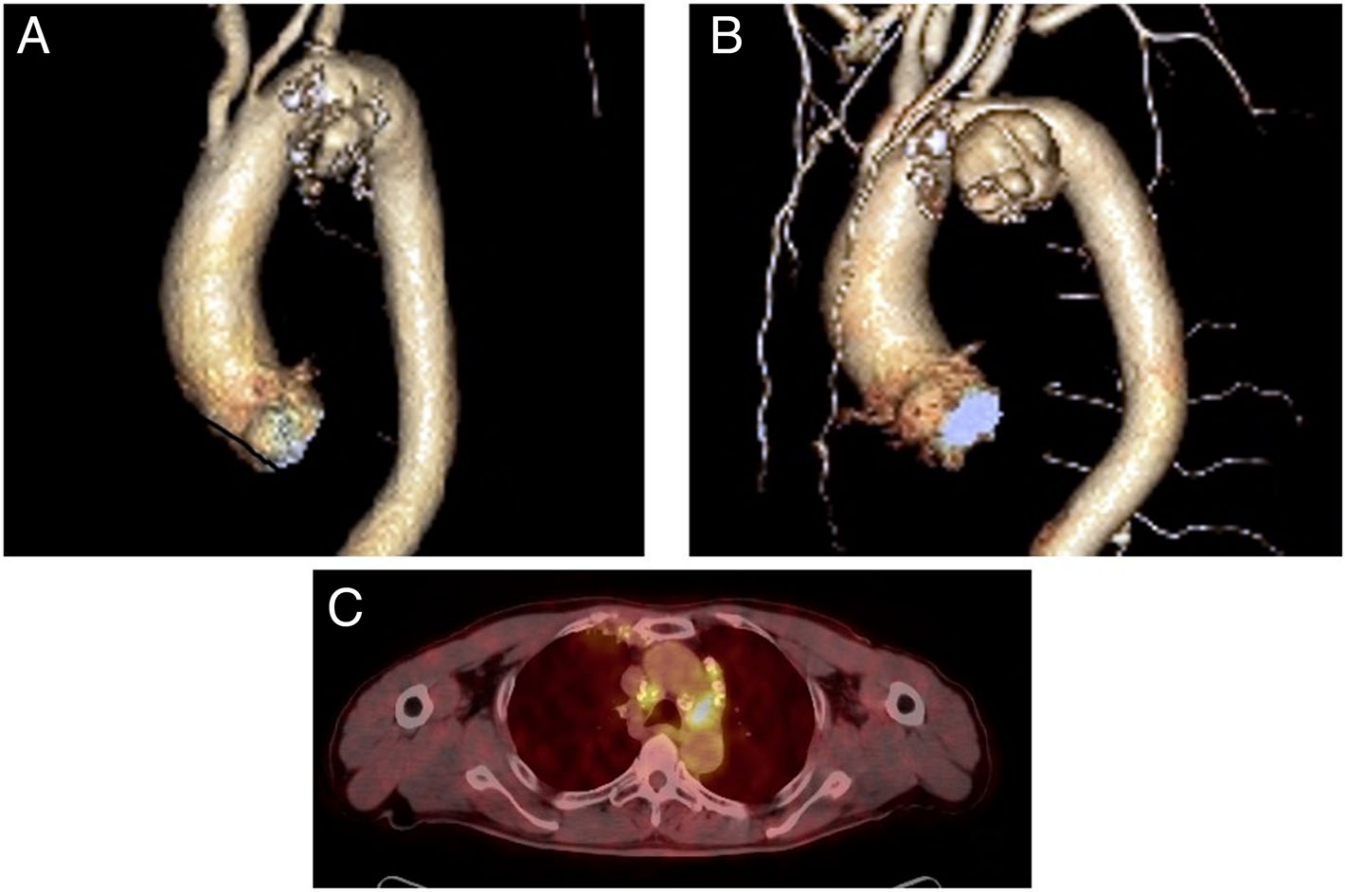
a Computed tomography (CT) of the chest revealed a new saccular aneurysm of the aortic arch. b After 1 month, follow-up CT showed a rapidly expanding saccular aneurysm of the aortic arch. c F-18 fluorodeoxyglucose positron emission tomography with computed tomography showing intense fluorodeoxyglucose avidity in soft tissue involving the aneurismal segment of the aortic arch
